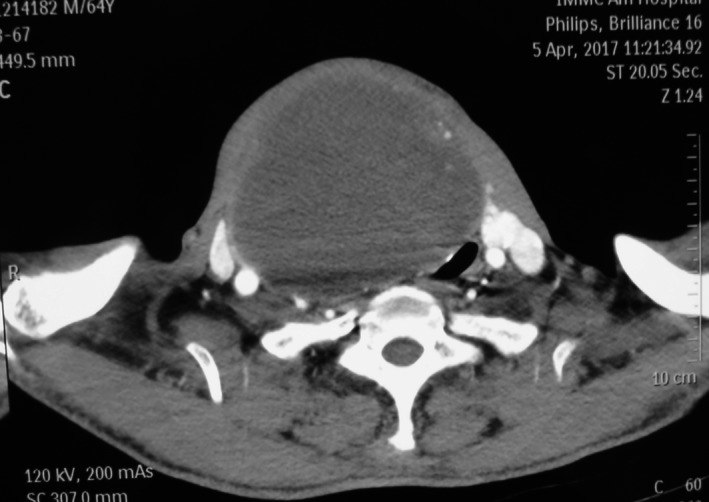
Computed tomography (CT) scan of the neck shows a homogeneous large mass in the right thyroid, causing compression of the trachea and the esophagus, and causing the tracheal stenosis
